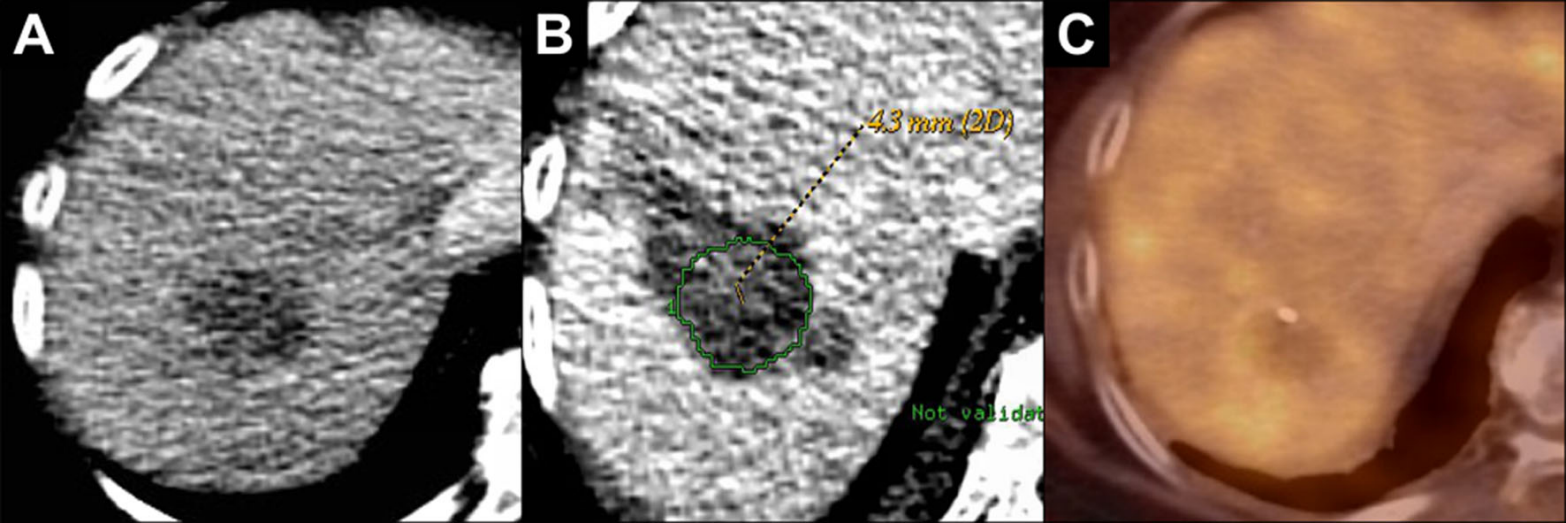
An 86-year-old woman with a metastasis of a colon carcinoma in segment VII of the liver and ablation without measurable margin. a The target lesion is seen as a hypodensity on ce-CT scan. b The segmentation of the target lesion projected in the registered liver 24 h after the ablation. The lesion is covered by the ablation zone, without a measurable margin (Category B). c The follow-up PET/CT after 9 months shows no FDG uptake around the ablation zone
